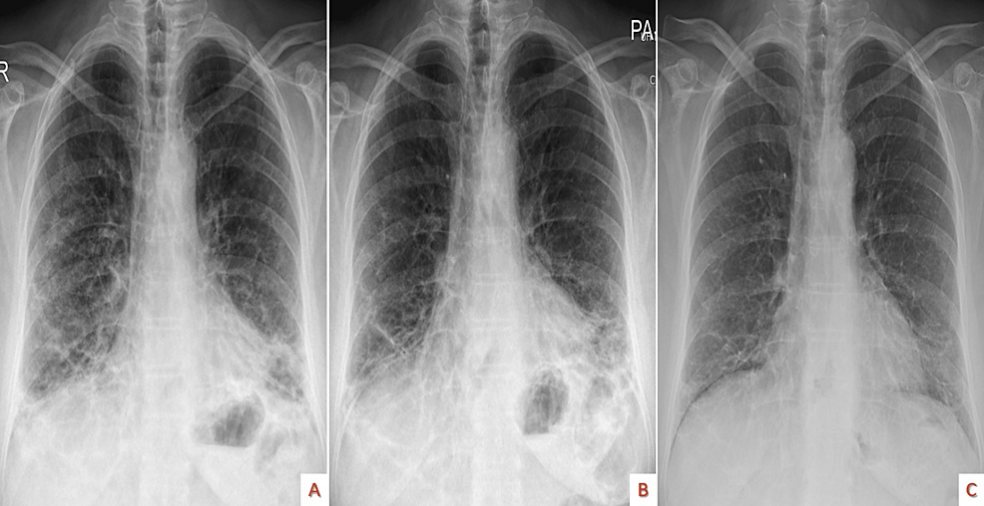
Serial inpatient follow up chest X-rays Radiological improvement with a decrease of reticulation and infiltrates, steroid naive (A), after four days of therapy (B), after eight days (C).
